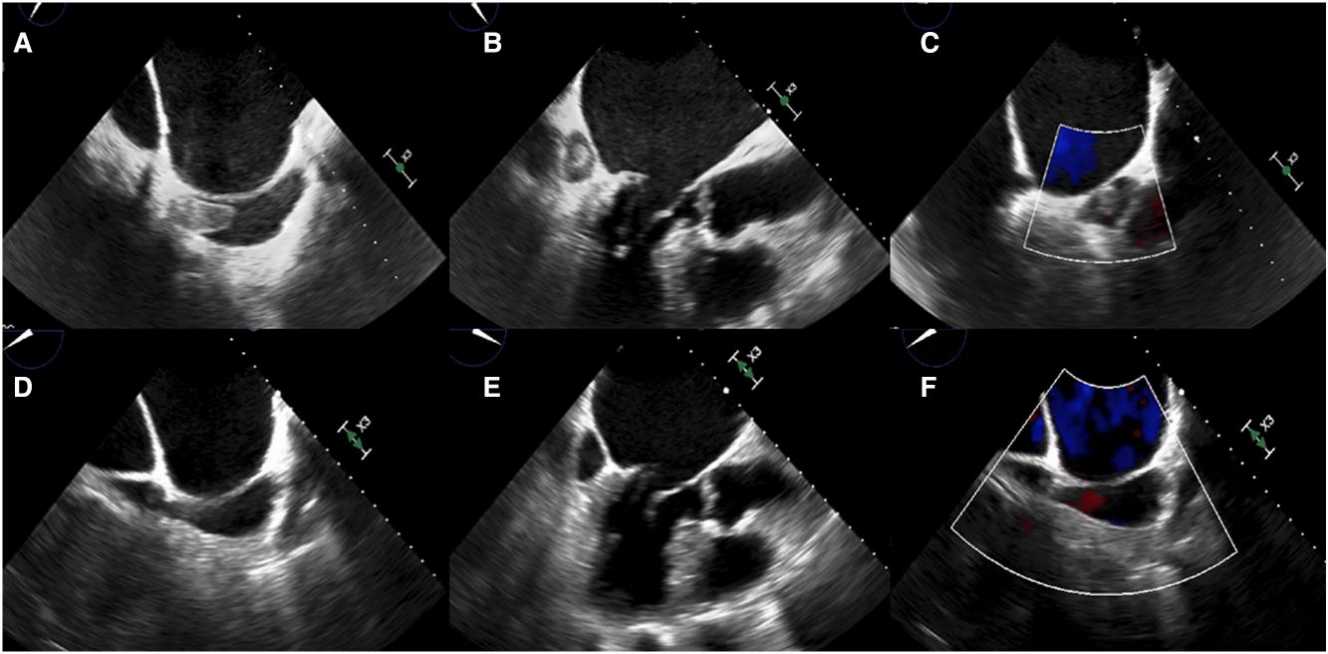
Transoesophageal echocardiogram. Transoesophageal echocardiogram showing thrombosis in the coronary sinus (*A–C*). New transoesophageal echocardiogram performed 4 weeks after admission that shows resolution of thrombosis with persistent residual sinus dilatation (*D–F*).
